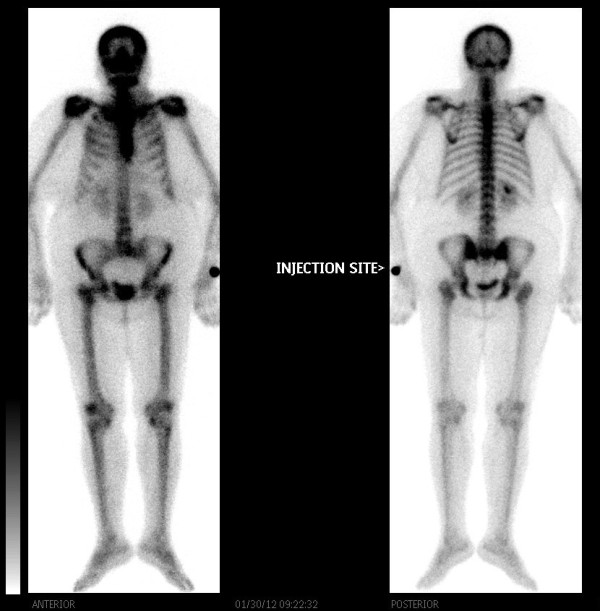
Bone scintigraphy showing no evidence of distant bone metastasis.
